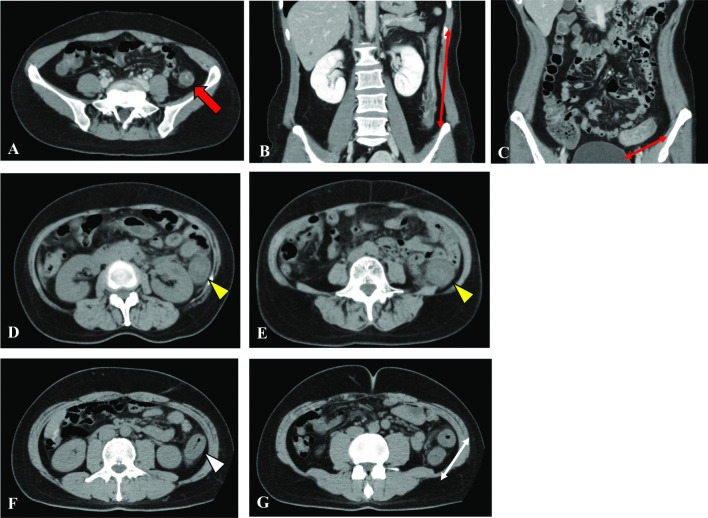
Representative computed tomography (CT) images from patients with COVID-19 and acute hemorrhagic colitis. A–C Axial and coronal CT images of a 45-year-old patient showing evidence of acute hemorrhagic colitis involving the descending to sigmoid colon appearing as thickening along with peri-colic fat stranding (red arrow and thin red arrows). D, E Axial CT images of a 59-year-old patient revealed acute hemorrhagic colitis involving the descending colon that showed marked thickening with peri-colic fat stranding (yellow arrowheads). F, G Axial CT images of a 39-year-old patient revealed acute hemorrhagic colitis involving the splenic flexure to descending colon that showed marked thickening with peri-colic fat stranding (white arrowhead and thin white double-headed arrow)
